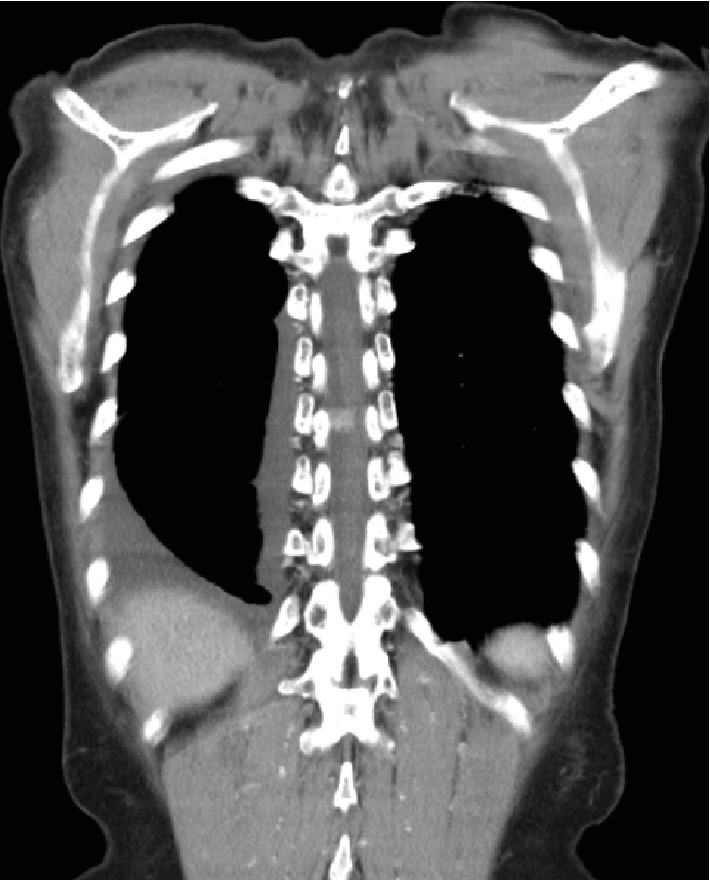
Follow-up CT with contrast medium one month after surgery for clear cell renal cell carcinoma showing a homogeneously enhanced mass without intralesional calcifications.
